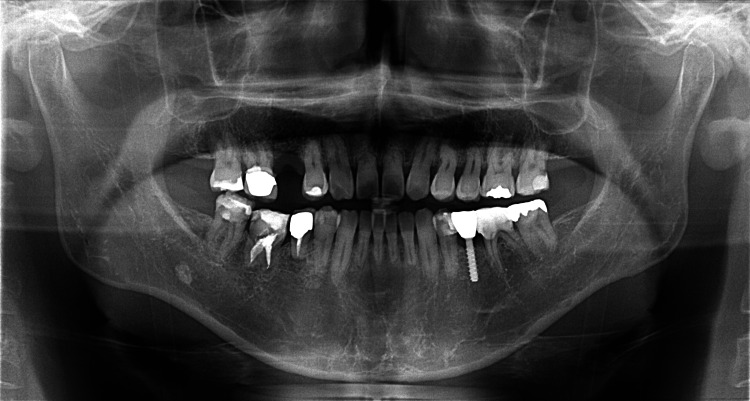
Panoramic radiograph three years after autotransplantation
